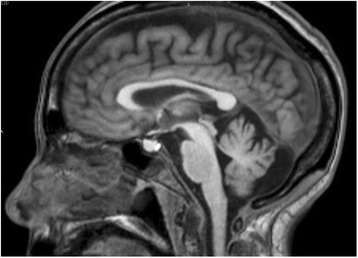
Manganese deposits in CNS structures. Coronal MRI T1 image showing hyperintensity signal in the midbrain as consequence of manganese deposition
